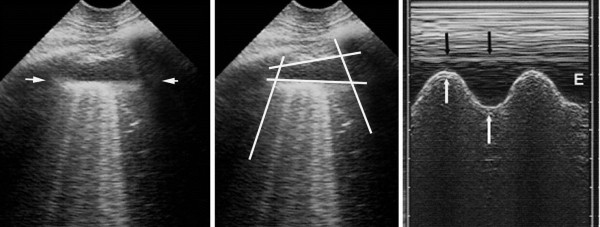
Pleural effusion. Left and middle: minute pleural effusion at the PLAPS-point. Below the pleural line, a line regular and roughly parallel to the pleural line can be seen: the lung line, indicating the visceral pleura (arrows). This line, together with the pleural line and the shadow of the ribs, display a kind of quad: the quad sign. Right: M-mode shows a movement of the lung line (white arrows) toward the pleural line (black arrows) on inspiration—the sinusoid sign, indicating also a free pleural effusion, and a viscosity enabling the use of small caliper needle if thoracentesis is envisaged. E, expiration. Quantitative data: this effusion found at the PLAPS-point has an expiratory thickness of roughly 13 mm, i.e., an expectedly small volume (study in progress). A 15-mm distance is our minimum required for safe diagnostic or therapeutic puncture, allowing to simplify the problem of modeling the real volume of an effusion (Ref. 14). Extract from “Whole body ultrasonography in the critically ill” (2010 Ed, Chapter 15), with kind permission of Springer Science.
